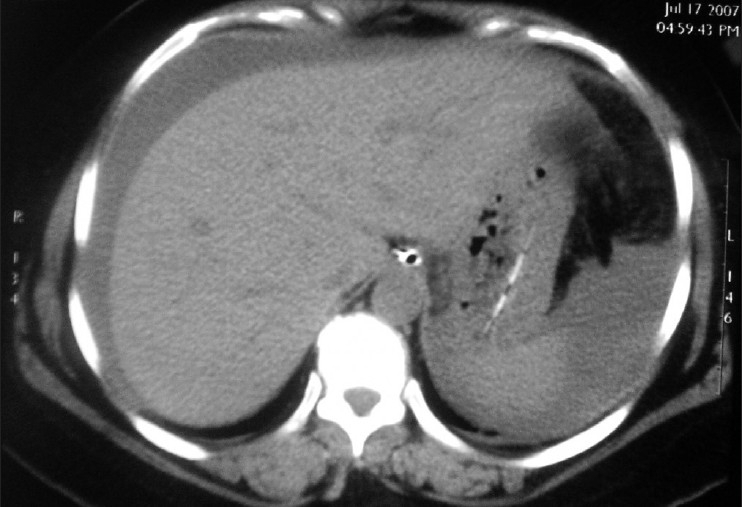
Sub-diaphragmatic fluid collection with a CT attenuation value of 30 suggesting hemoperitoneum
